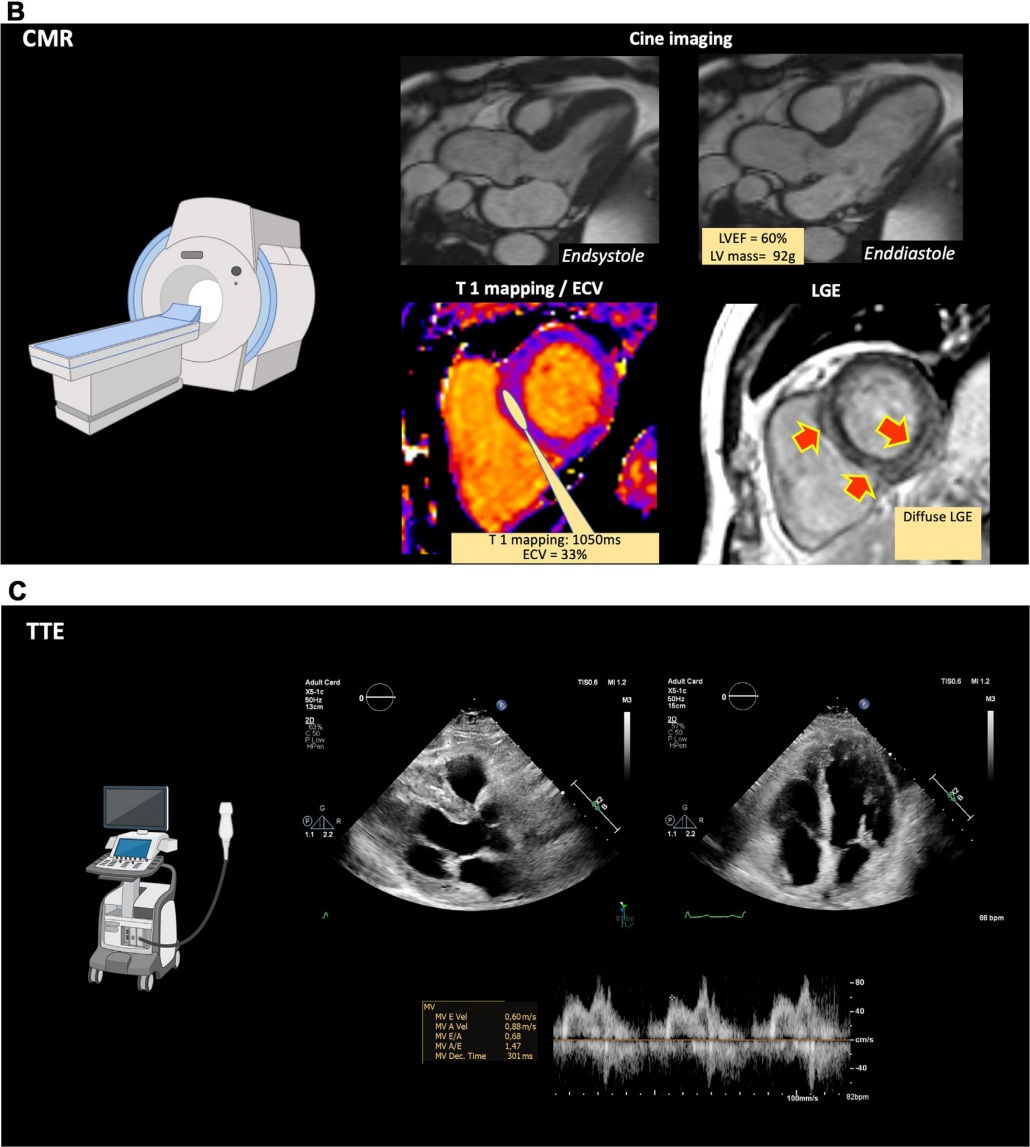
Multimodality Cardiac Imaging Findings Results from multimodality cardiac imaging investigations. (A) Scintigraphy using technetium-99m–labeled 3,3-diphosphono-1,2-propanodicarboxylic acid (^99m^Tc-DPD) showing myocardial tracer uptake consistent with a grade 2 as described previously by Perugini.^2^ The standardized maximal uptake value at 1 hour (SUVmax) depicted in the upper right of the figure provides a quantitative assessment of cardiac amyloid burden and has previously been described to correlate well with measures of tissue characterization on cardiovascular magnetic resonance (CMR).^3^ Axial single-photon emission computed tomography/computed tomography image showing tracer uptake in the myocardial septum. (B) Upper row depicting 3-chamber views obtained from CMR imaging in end-diastole and end-systole with normal left ventricular ejection fraction (LVEF) and left ventricular mass (LV mass). Bottom panel presenting the findings from tissue characterization methods. Assessment of parametric mapping (T1) and calculation of extracellular volume (ECV) revealed elevated T1-values and mildly elevated ECV. Late gadolinium enhancement (LGE) showing sub-endocardial and epicardial diffuse enhancement consistent with amyloid fibril deposition. (C) Representative images of parasternal long axis (upper-left pane) and 4-chamber (upper-right pane) views on transthoracic echocardiography. Pulsed wave Doppler measurements of inflow velocities across the mitral valve showing abnormal diastolic relaxation with a ratio of early and late diastolic filling across the mitral valve of 0.68 and a prolonged mitral E-wave deceleration time of 301 milliseconds.
